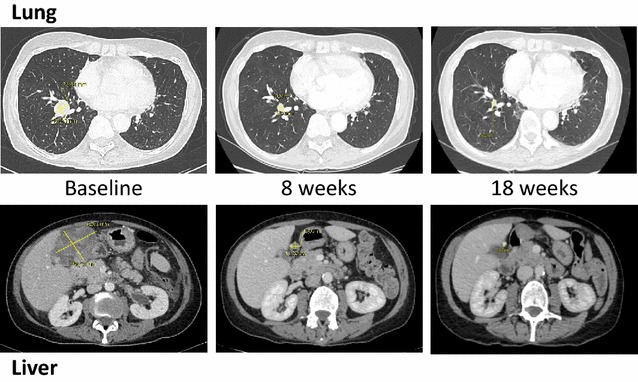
Representative computed tomographic images demonstrating treatment efficacy. Lesions in lung and under liver are presented at baseline and after 8 and 18 weeks of treatment with a combination of BRAF and MEK inhibitors
